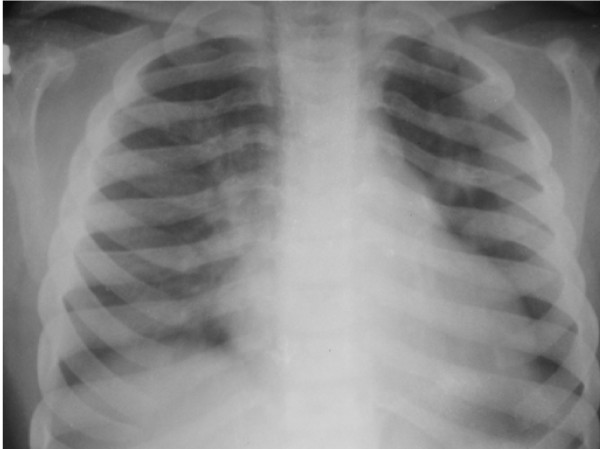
Anteroposterior chest X-ray showing paddle and/or spatulated ribs.
